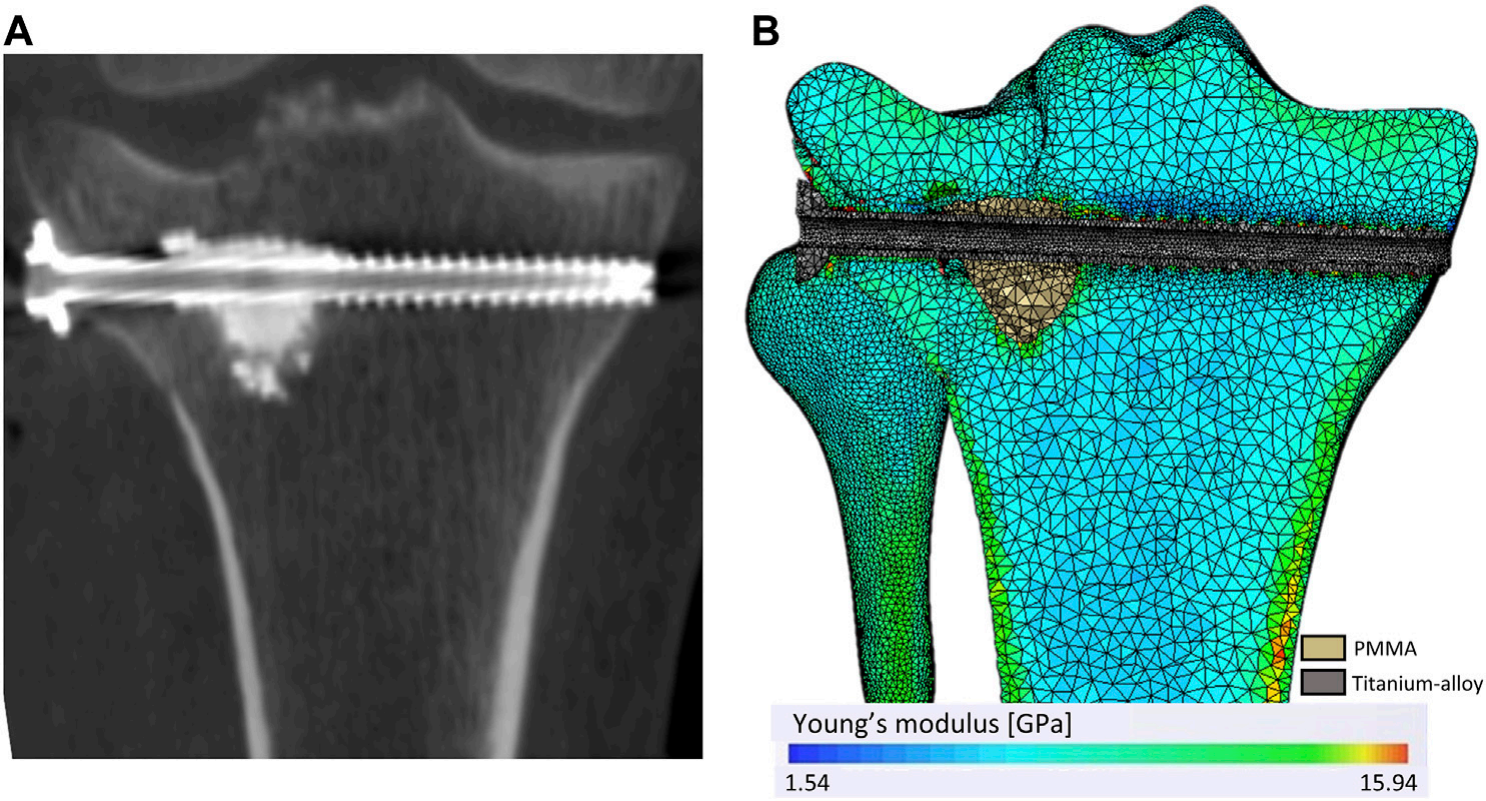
Mesh and heterogeneous distribution of bone material properties. (A) A coronal plan of the 3D X-ray image. (B) The mesh and the material properties distribution according to the bone density observe through the 3D X-ray image.
